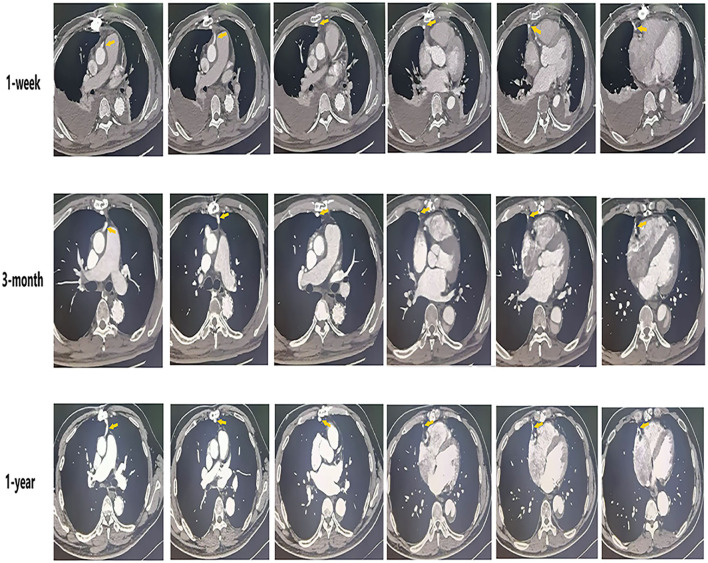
The condition of SVG-AO grafts at different times (coronary CT angiography).
